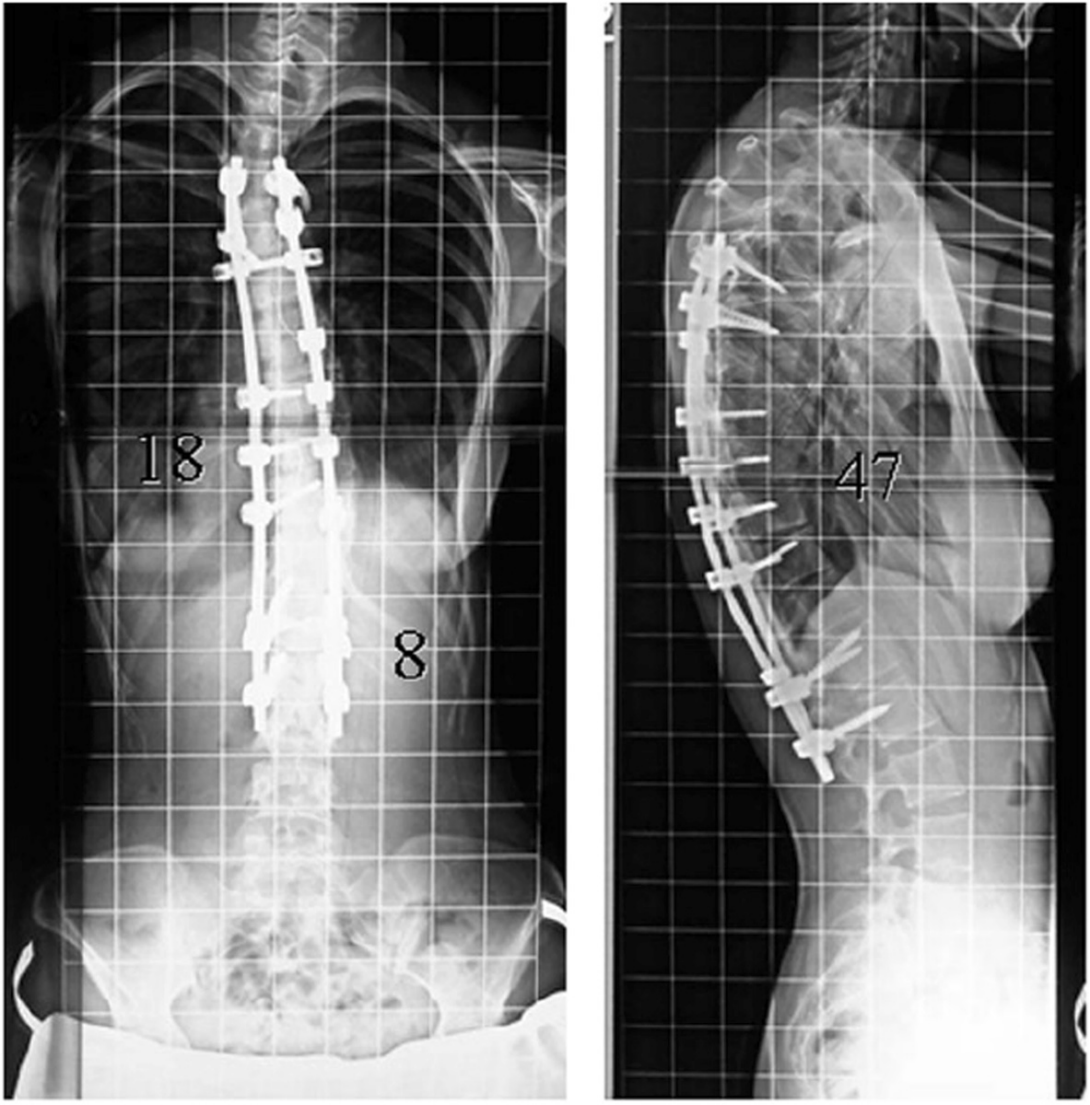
Radiographs after reinstrumentation surgery.
